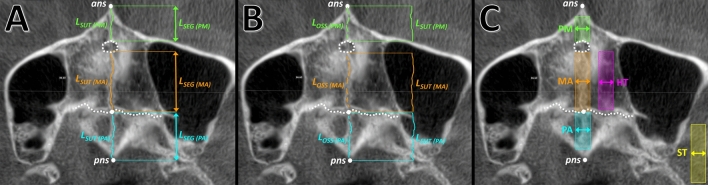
Description of the anatomical assessment of the midpalatal suture on CBCT images. Anterior nasal spine (*ans*), posterior nasal spine (*pns*), incisal canal (dashed circle), and posterior transverse suture (dashed line) are indicated in white. Suture length (*L*_*SUT*_) and segment length (*L*_*SEG*_) (A). Suture length (*L*_*SUT*_) and ossified suture parts (*L*_*OSS*_) (B). Areas in which the grey density was measured for representing the hard tissues (HT), and the soft tissues (ST), arrows indicate a 4.0 mm width (C). Measurements are shown in different colours for each suture segment: premaxilla (PM), maxilla (MA), and palatine (PA).
